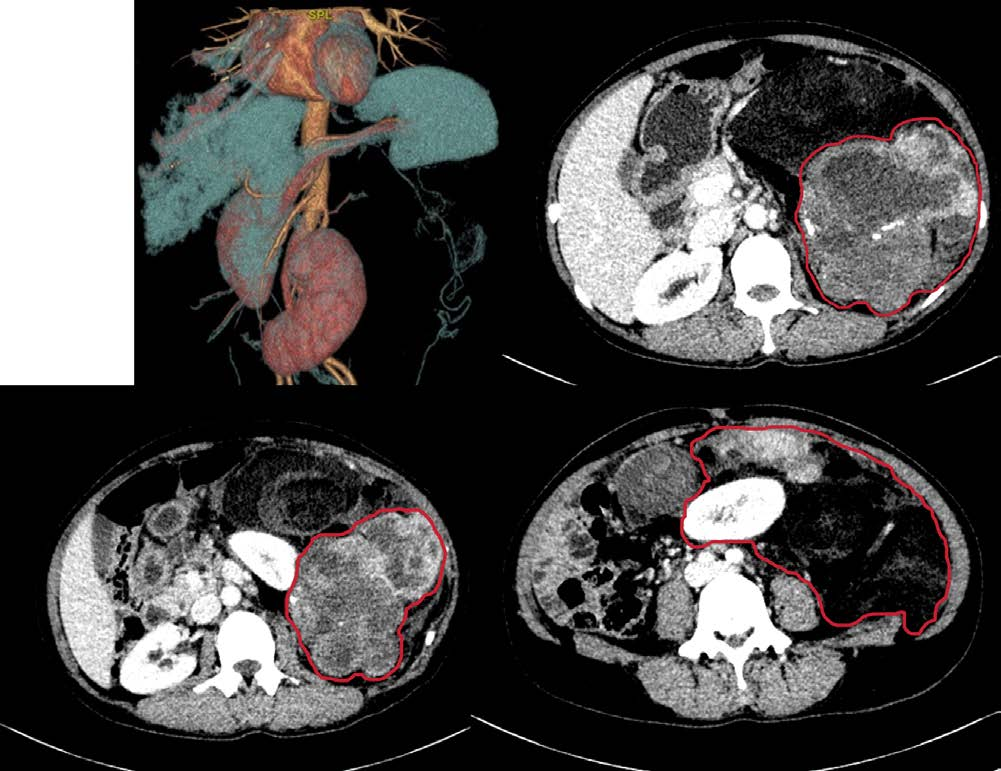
Contrast-enhanced CT. CT = computed tomography.
